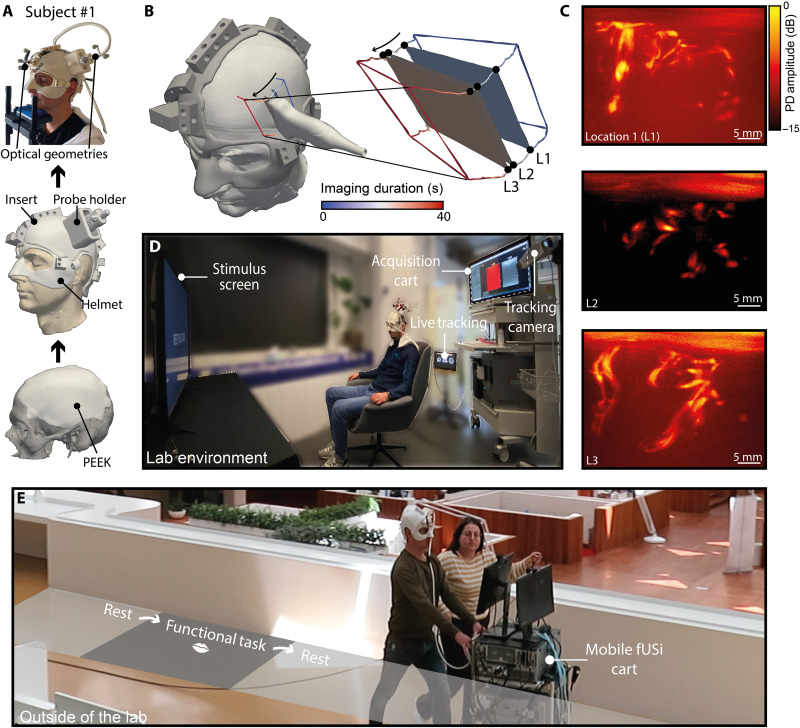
Key components of our experimental setup. (A) To facilitate stable and reproducible fUSi throughout the experiment, we designed a custom 3D-printed helmet based on the subject’s MRI. The helmet consisted of a probe insert, which allowed us to revisit the same 2D plane over time, positioned over brain ROIs for functional tasks. Optical geometries were attached to the helmet and probe to monitor their relative position, facilitating fUSi-MRI coregistration and offline 3D reconstruction of the ultrasound path. (B) Example of an ultrasound path as reconstructed using our offline optical tracking data. In this case, the probe was moved over the subject’s head using a linear translation (see the directions of arrows). Three interesting imaging locations in terms of vasculature (L1 to L3) were marked within this path, as examples to showcase. (C) power Doppler images (PDIs) of interesting brain vasculature seen at three showcase locations (L1 to L3), as marked in (B). A full overview of the vascular images acquired within this path can be found in movie S1. (D) Overview of the experimental setup in our laboratory environment. The subject was placed in front of a screen showing the stimulus. The equipment, including our tracking camera, our custom fUSi acquisition cart, and our live tracking tool based on our optical tracking, is also displayed. (E) Example of an experimental acquisition using our mobile fUSi cart outside of the laboratory environment. The cart was pushed by the subject himself while performing the task. Permission was required from the subject to publish images.
